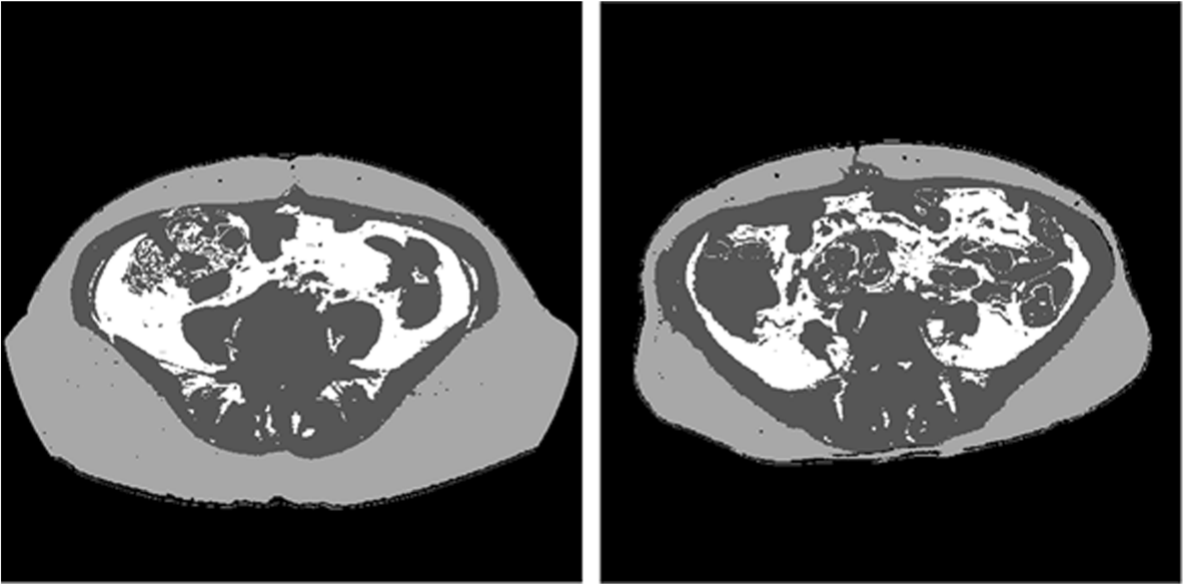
Two examples showing the segmentation of VFA and SFA in four CT image slices. In these two images, SFA is shown in *light gray* color, VFA is represented by *white* color, and *dark gray* color masks other human organs and/or structures
